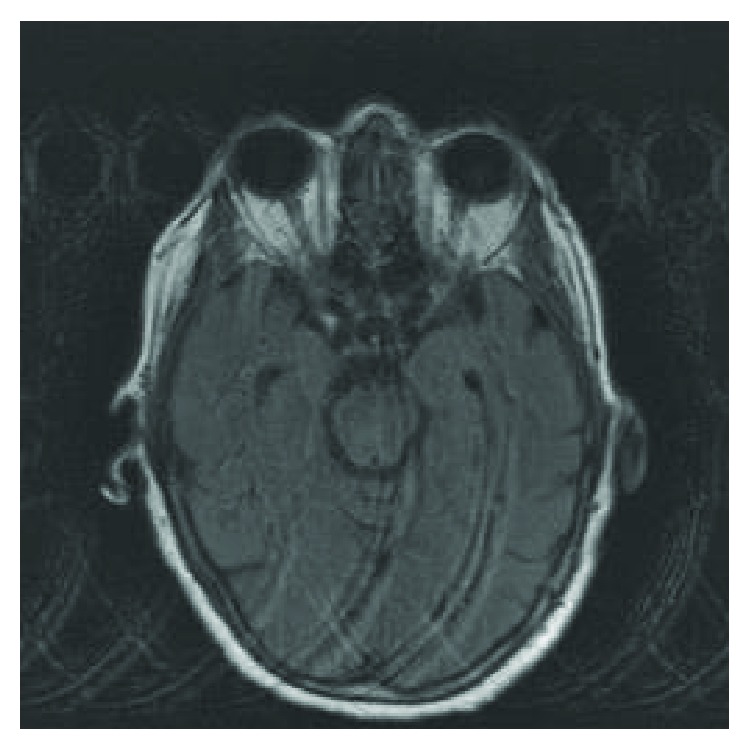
Axial T2-weighted sequence through the midbrain.
